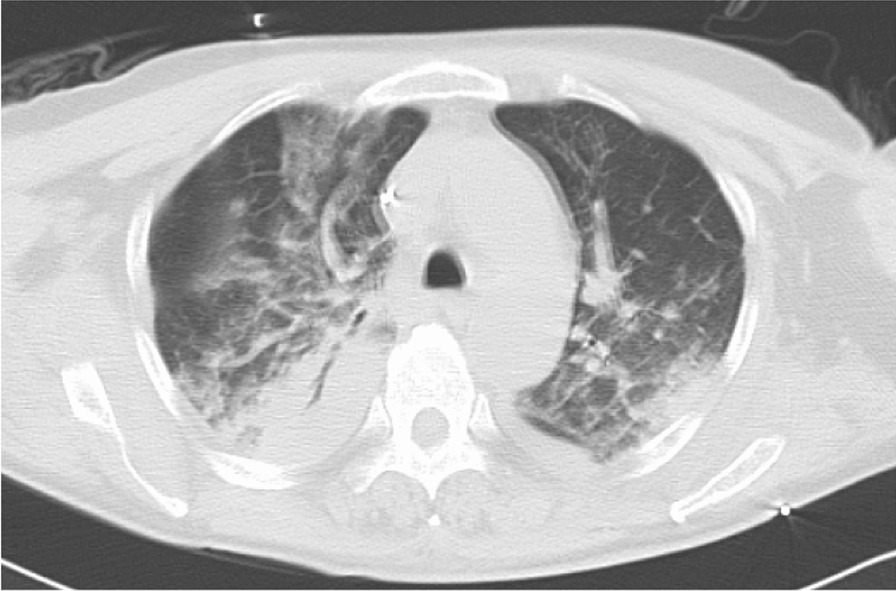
Chest computed tomography showed infiltration and bronchial thickening of right upper lobe
